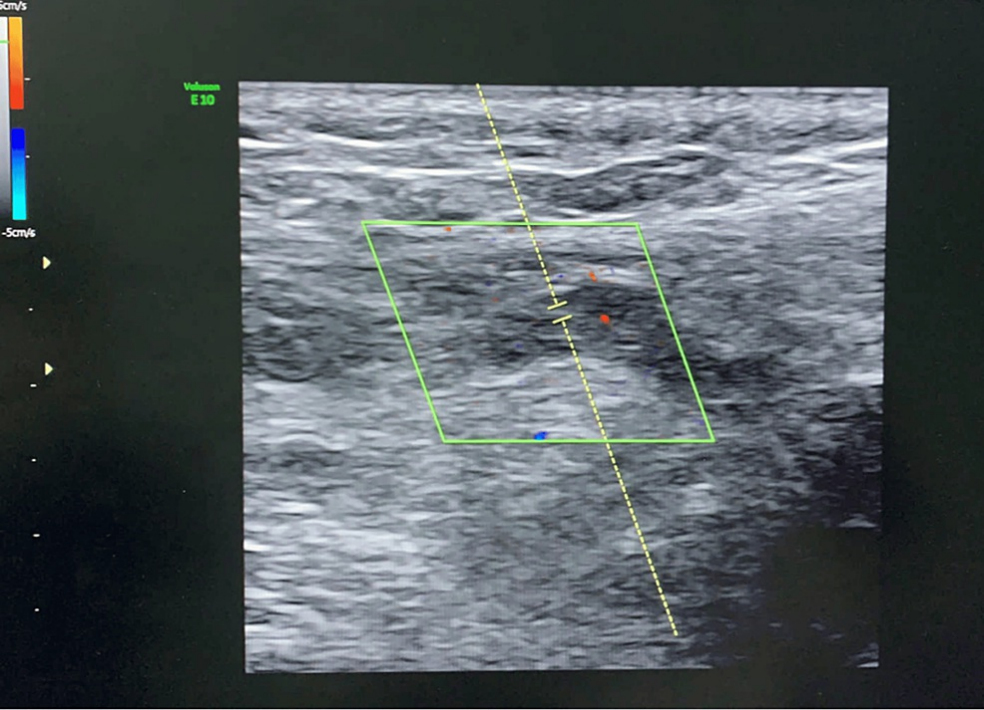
Left scrotal Doppler ultrasound revealed a dilated vein in the left spermatic cord.
